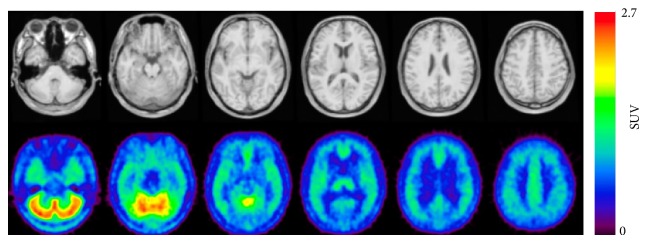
PET/MRI images of [^11^C]ITMM (40–60 min) obtained from 5 healthy human subjects. Upper: MR image and lower: averaged [^11^C]ITMM PET image [85].
